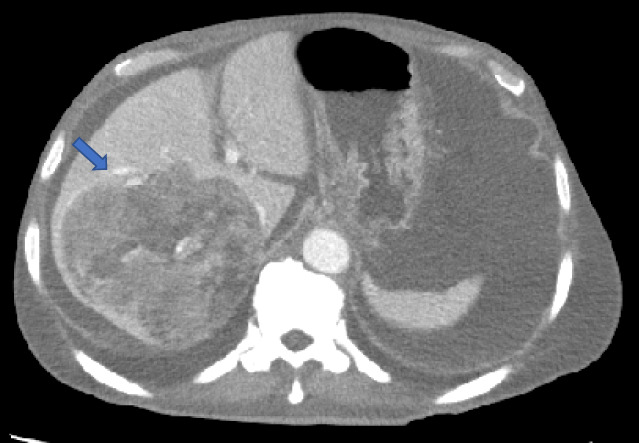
Enhanced computed tomography revealing hepatic metastases rupture with substantial hemoperitoneum hematemesis on the 14th day of the fifth course of upfront docetaxel.
